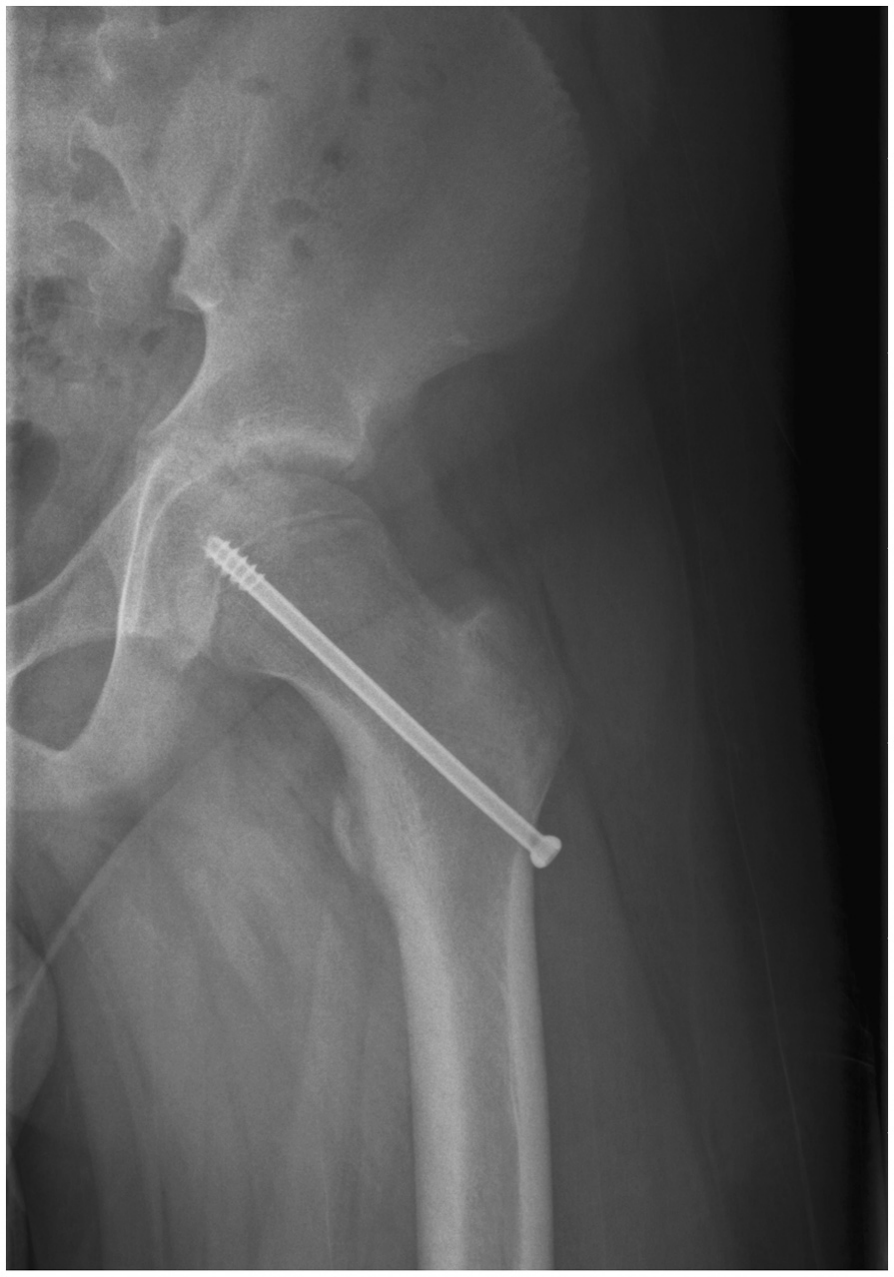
Patient 1. The X-ray of the left hip after *in situ* fixation.
